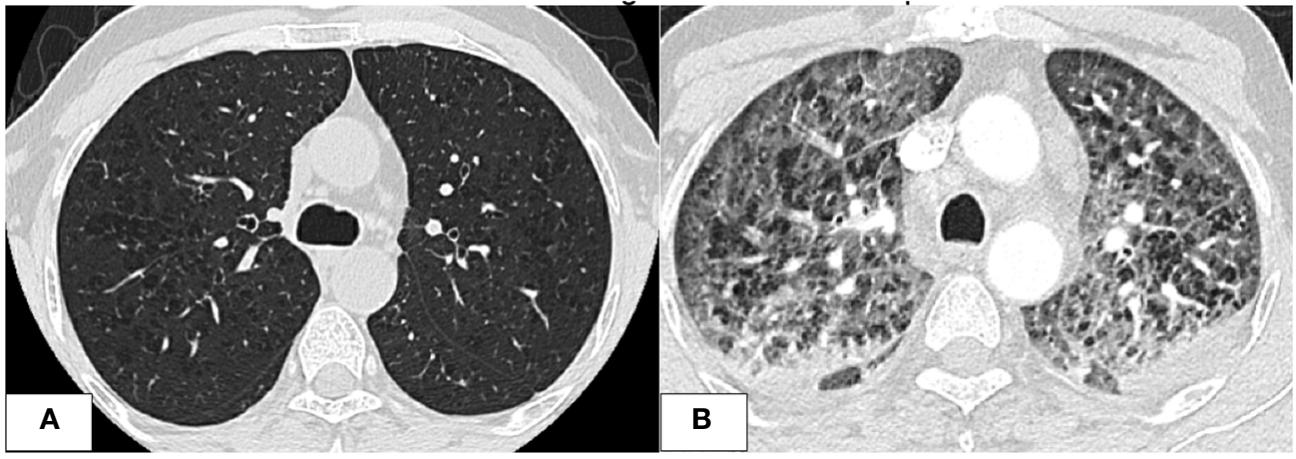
CT chest images. (A) After the first cycle of azacitidine, at that time, the patient was treated for invasive fungal infection. Imaging shows significant improvement of previous bilateral ill-defined nodular opacities with residual GGOs and micronodules. (B) Imaging was done at the time of the development of respiratory symptoms after the second cycle of azacitidine. Imaging showed worsening diffuse GGOs with smooth interlobular septal thickening and centrilobular nodules with bilateral bronchial wall thickening and small bilateral pleural effusions.
